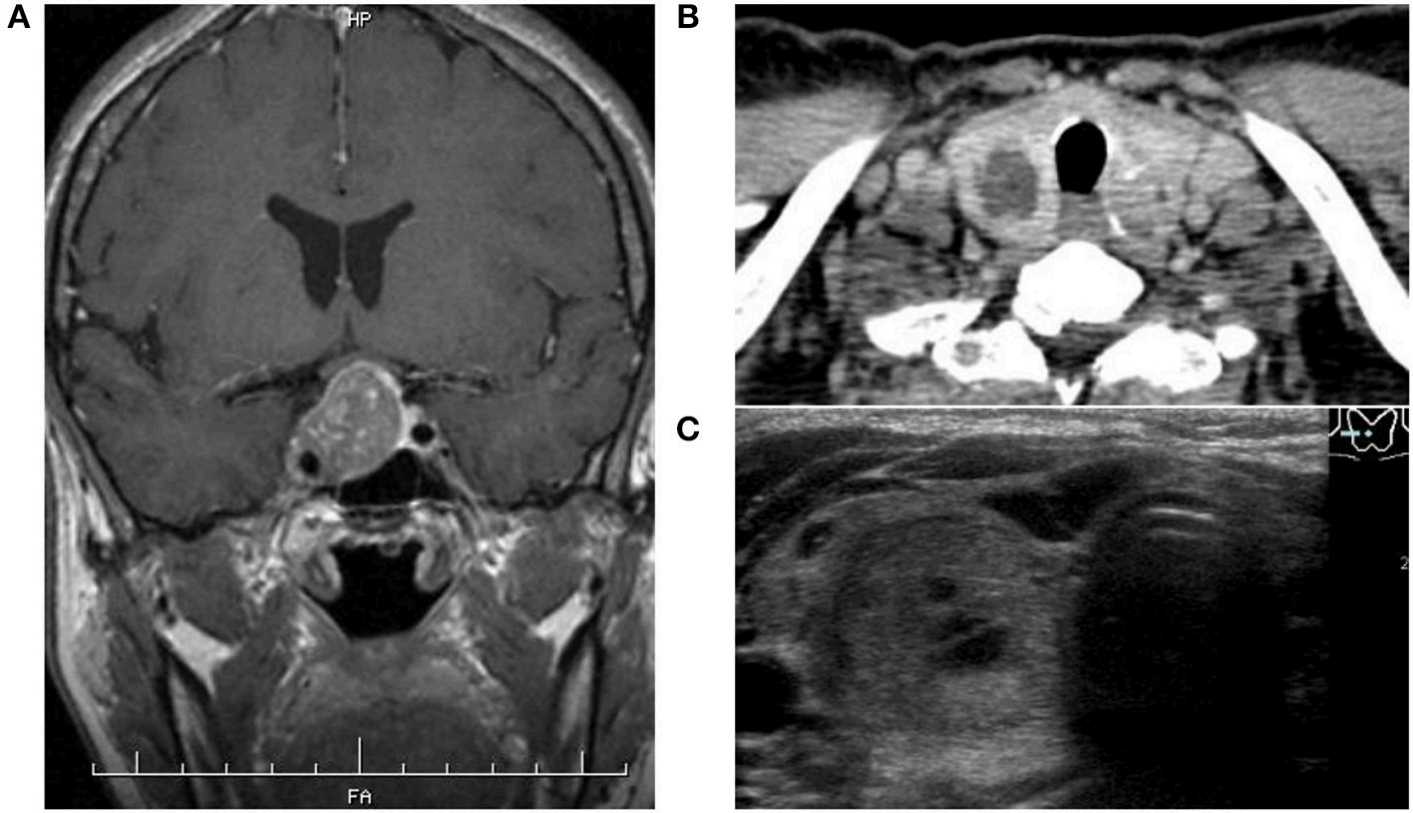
(A) Magnetic resonance imaging showed a pituitary tumor demonstrated less enhancement than the normal pituitary gland after intravenous contrast administration with invasion of the right cavernous sinus. (B,C) Thyroid enhanced computed tomography and ultrasonography showed several nodules inside the thyroid gland, of which the largest located at the bottom of the right lobe contained a cystic component and high vascularity.
